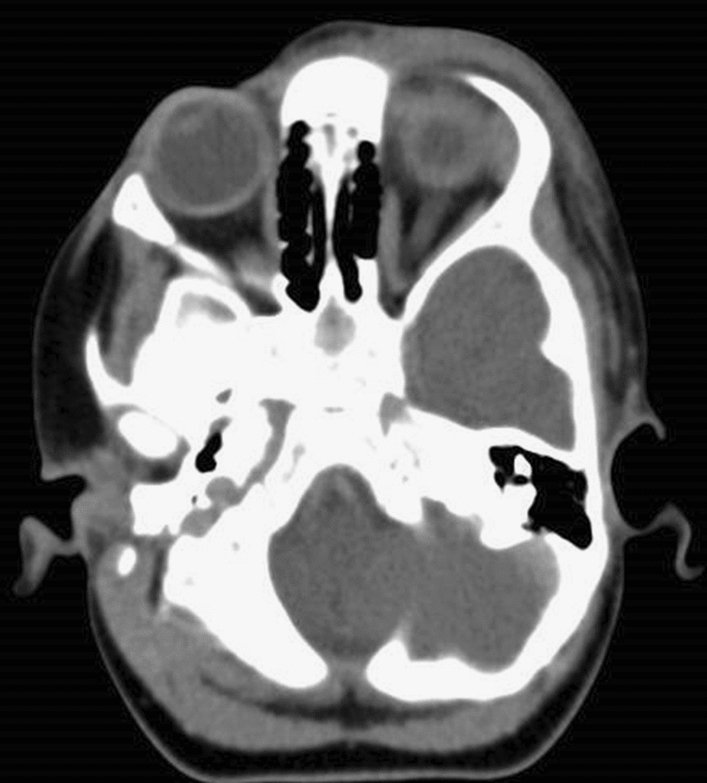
CT imaging of periorbital Molluscum abscess.
